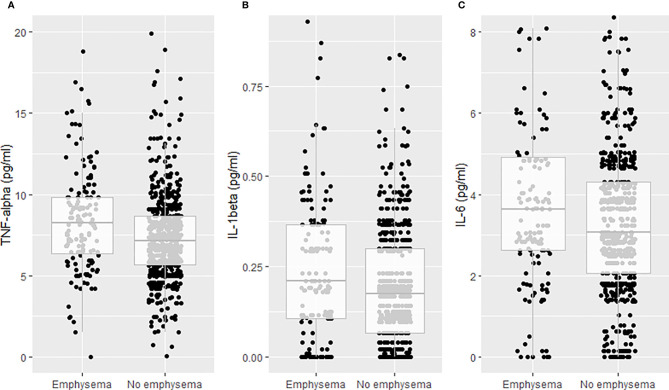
Plasma concentrations of tumor necrosis factor-alpha (TNFα), interleukin-1 beta (IL-1β) and interleukin-6 (IL-6) by radiographic emphysema. (A) TNFα. (B) IL-1β. (C) IL-6.
